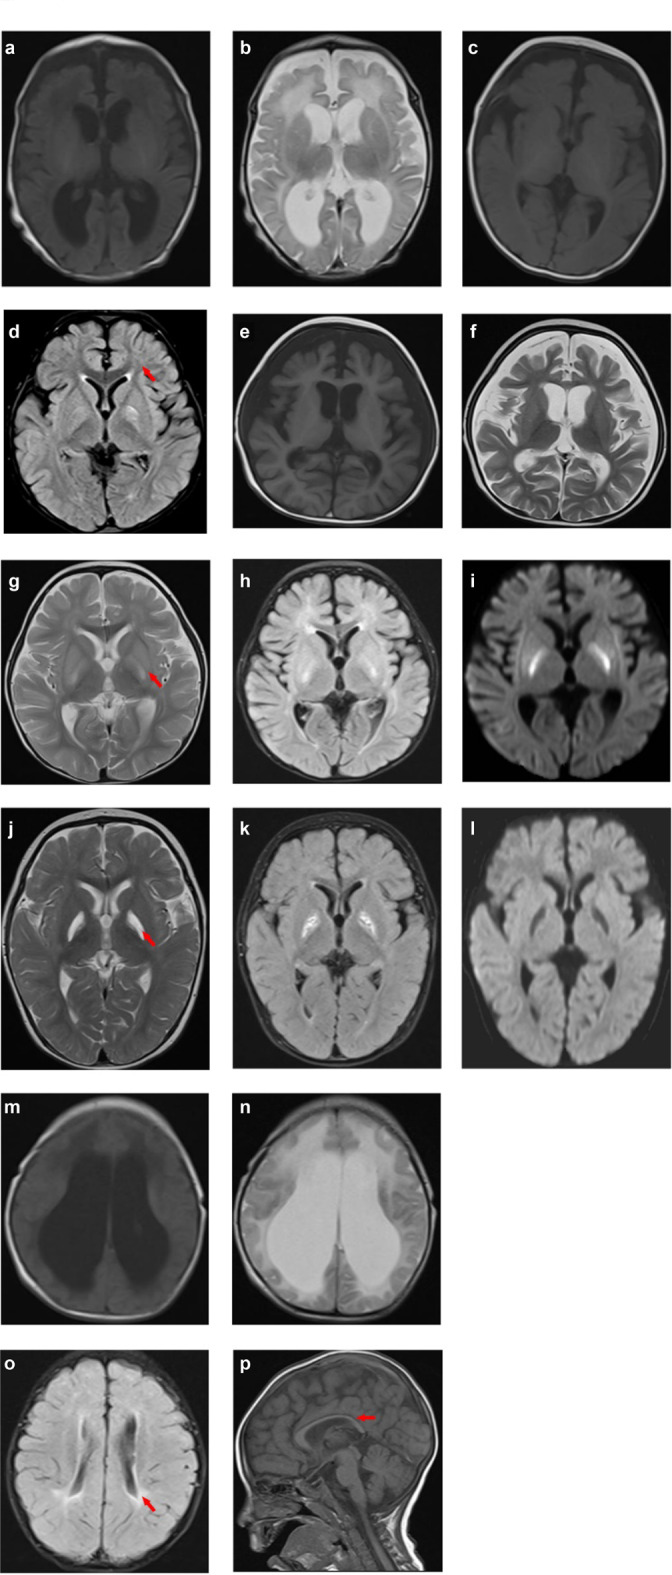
Brain MRI findings. (a–b) The MRI findings of ventricular dilation were acquired with axial T1-weighted image (a) and axial T2-weighted image (b). (c) The MRI of a 4-month-old boy revealed myelination delay, with relatively weak hyperintensity in the internal capsules in the axial T1-weighted image. (e–f) The MRI images of cerebral atrophy were acquired with axial T1-weighted image (e) and axial T2-weighted image (f). (g–l) The MRI findings revealed basal ganglia changes (g–i) and globus pallidus changes (j–l), and the axial T2-weighted (g, j) and T2-FLAIR (h, k) images showed symmetric hyperintense signals; the diffusion-weighed images showed high (i) and low signals (l), respectively. (m–n) The MRI findings of hydrocephalus were acquired with axial T1-weighted image (m) and axial T2-weighted image (n). (o) The axial T2-weighted MRI showed periventricular white matter abnormality. (p) The sagittal T1-weighted showed thin corpus callosum. MRI, magnetic resonance imaging
